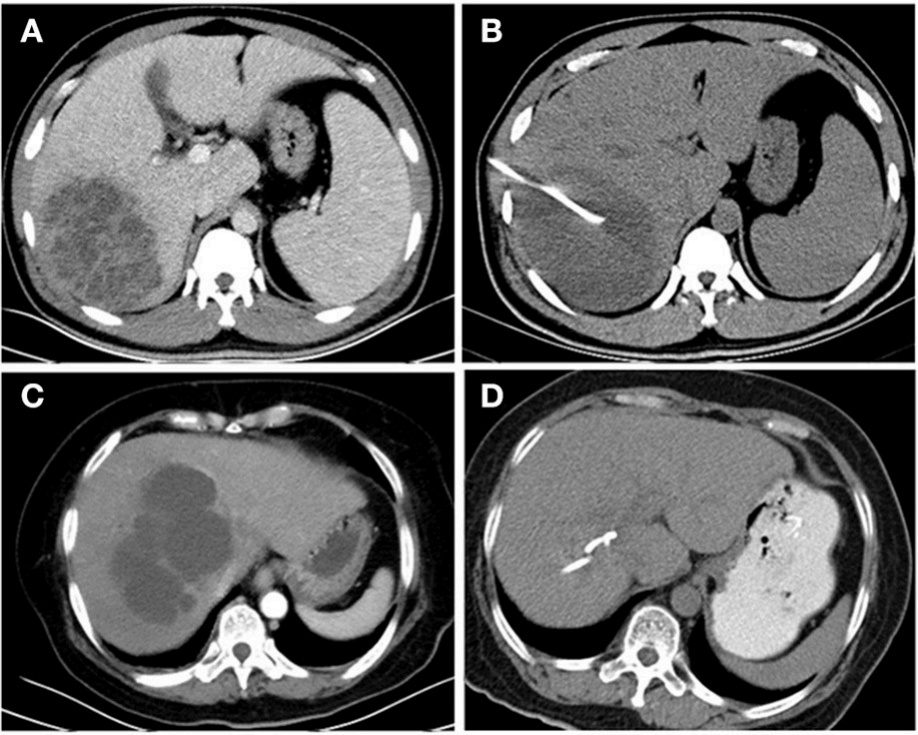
CT images of the livers of *K. pneumoniae* liver abscess patients before and 1 week after drainage. A 36-year-old man with a *K. pneumoniae* liver abscess (A). The diameter of the abscess did not decrease 1 week after drainage (B), and the abscess was thus defined as a drainage-resistant *K. pneumoniae* liver abscess (DRKPLA). A 62-year-old woman with a *K. pneumoniae* liver abscess (C). The abscess had almost disappeared 1 week after drainage (D) and was thus defined as a drainage-sensitive *K. pneumoniae* liver abscess (DSKPLA).
